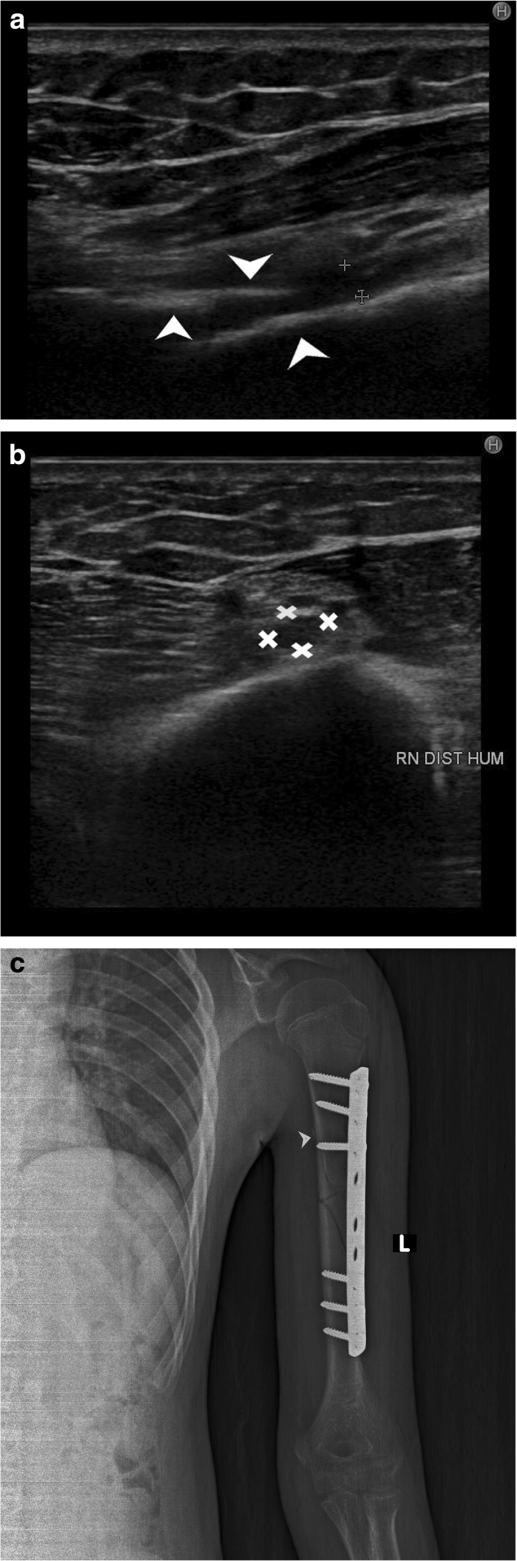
a Hypoechoic radial nerve is seen in the longitudinal plane (between crossheads) entrapped between two edges of the fracture segment (arrowhead). b Hypoechoic radial nerve, crossheads, in its short axis just distal to the site of entrapment. c Plain radiograph after open reduction and internal fixation in a patient with fracture of the humerus shaft (arrowhead). The nerve was impinged within the fracture segment in the spiral groove
